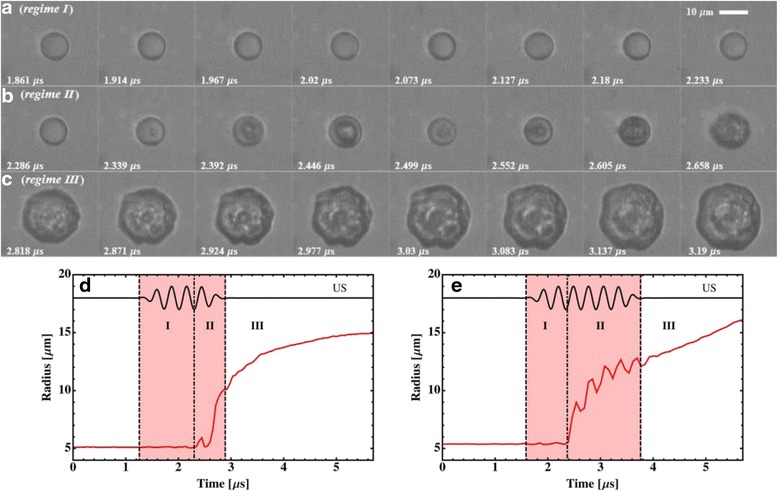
a–c A set of consecutive images showing acoustic droplet vaporization taken at 18.5 Mfps at an ambient temperature of 41 °C in three distinct regimes by 10-cycle ultrasonic pulse at a frequency of 3.5 MHz and peak negative pressure of 4.5 MPa and d, e two typical examples of the time dependent curves of the outer radius of the droplet/bubble complex obtained from the vaporization at ambient temperature of 41 and 50 °C, respectively, with courtesy of [24]
